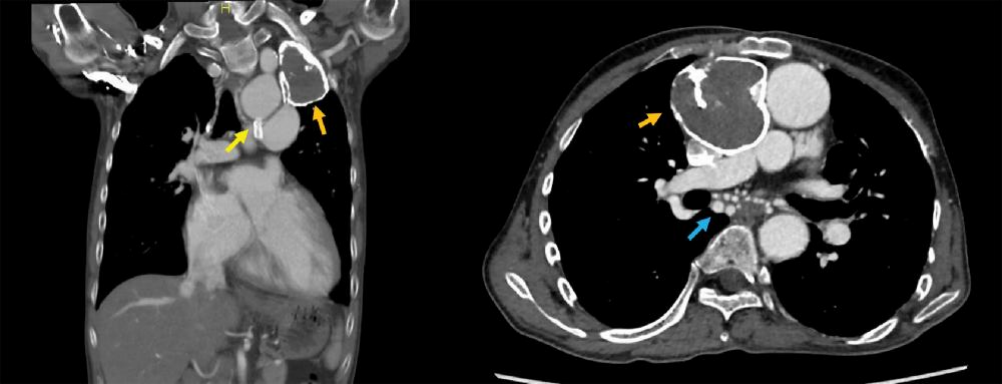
Contrast computed tomography showing a univentricular heart (double-inlet left ventricle), atrial septal defect, seroma formation (orange arrow), and patent ductus arteriosus (yellow arrow). Collateral vessel formation in the mediastinum is also increased (blue arrow).
